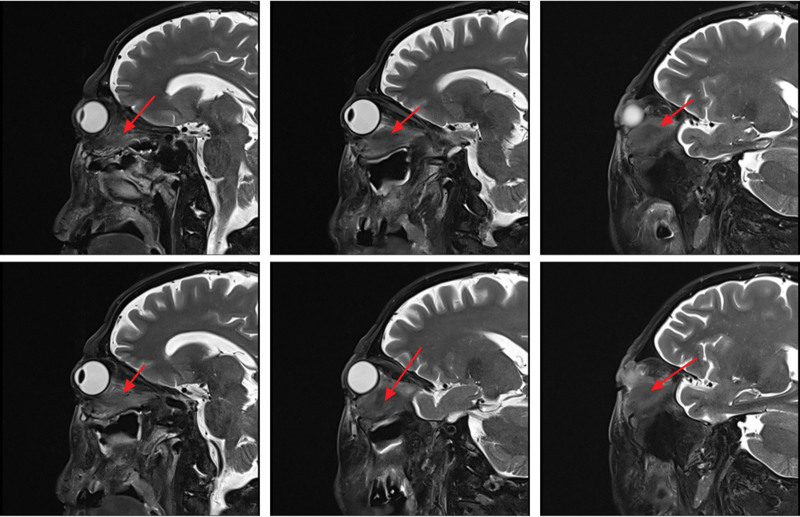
The patient MRI sagittal T2 phase showed slightly high signal (red arrows) in the left posterior fat space, left lateral eyeball, and left inferior muscle space mass. MRI = magnetic resonance imaging.
